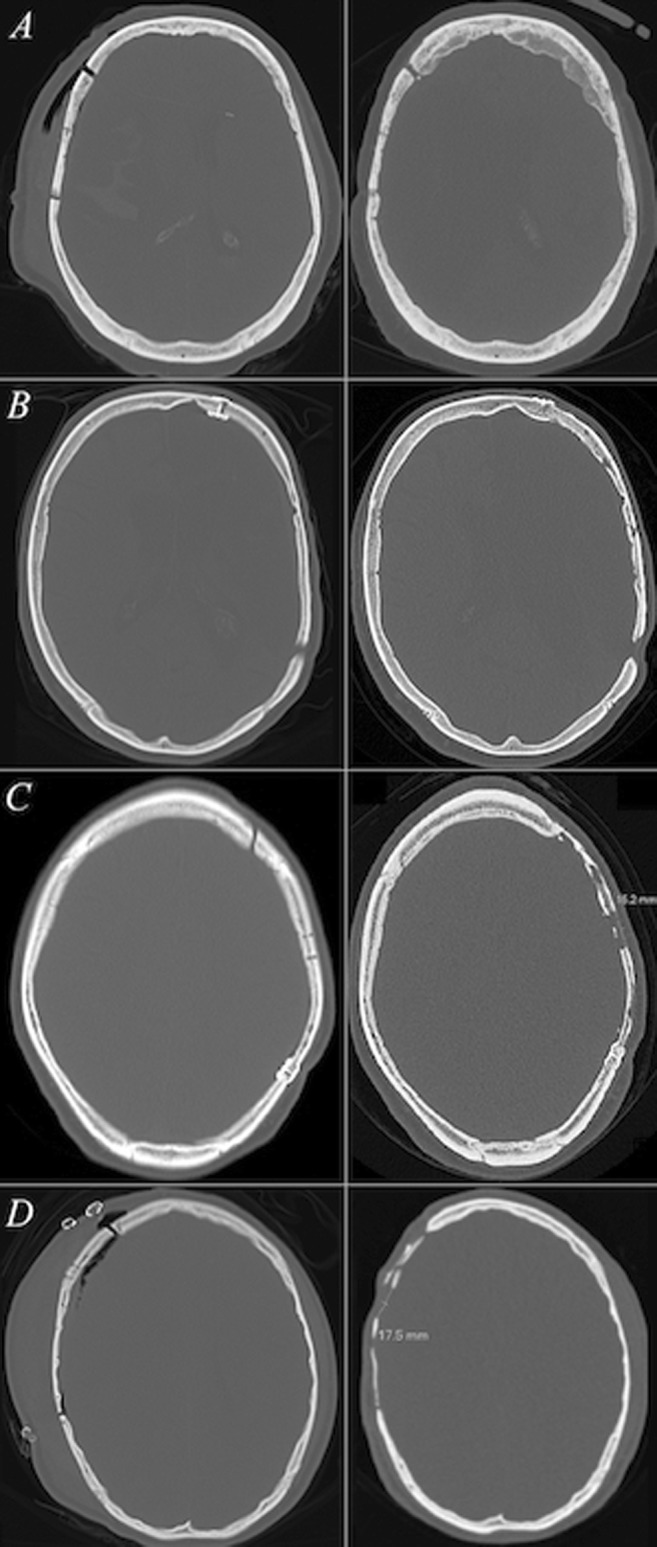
CT slices with bone window settings depict the initial postoperative and follow-up bone flap statuses (left and right columns, respectively) of four cranioplasty patients with different levels of bone flap resorption. Each row comprises one patient. In a, the follow-up bone flap status was given a mean Oulu resorption score of 0 (grade 0) as no signs of bone flap resorption were visible. The bone flap status of the patient in b was given a mean Oulu resorption score of 1.33 (grade I)—though the follow-up bone volume remained ≥ 75%, relatively mild bone flap resorption manifesting as multiple non-perforating cortical irregularities on both sides of the autograft was visible. More notable signs of bone flap resorption are depicted in c, where the follow-up status of the cranioplasty was graded a mean Oulu resorption score of 8 (grade II). The postoperative bone volume had decreased to 25–75% of the original volume along with diffuse resorptive changes on the bone flap area and a bicortical perforation exceeding 1 cm in diameter. Yet, the autograft had remained somewhat fixed to the cranium. In d, the cranioplasty had failed and the mean Oulu resorption score was 9 (grade III). The follow-up bone flap volume was < 25% of the original, bicortical perforations larger than 1.0 cm were noted and the resorptive changes within the bone flap were diffuse. Neither of the surgeons recommended further interventions for patients a or b. For the patient c, one surgeon recommended a neurosurgical consultation and for the patient d, both surgeons recommended consultation of a neurosurgeon and additionally a re-cranioplasty evaluation due to bone flap failure
